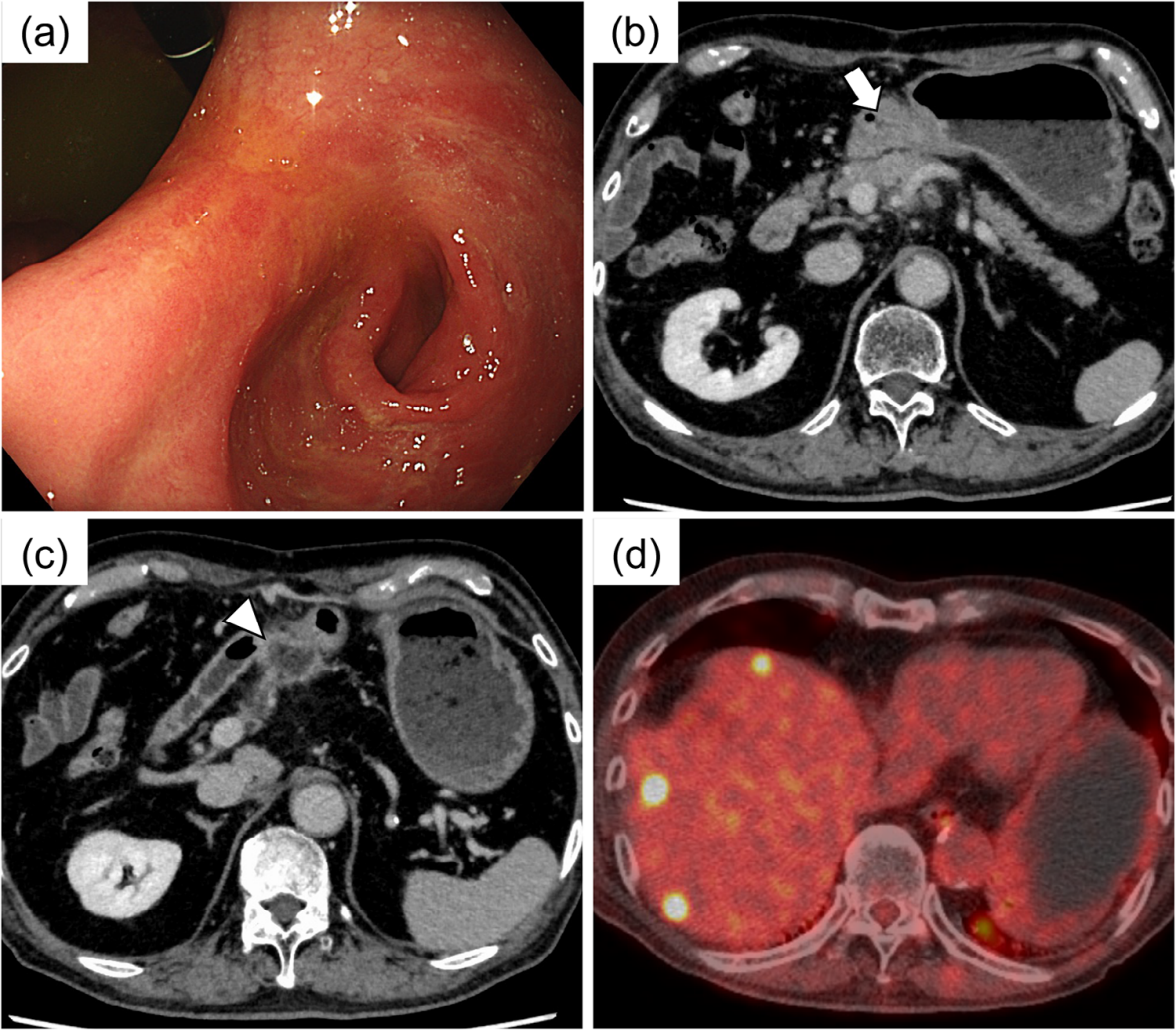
Endoscopic findings, computed tomography (CT), and 18F‐fluorodeoxyglucose positron emission tomography (FDG‐PET)/CT images. (a) Esophagogastroduodenoscopy (EGD) showing a singular ulcer scar. (b, c) CT revealing wall thickening of the gastric antrum (white arrow) and an irregular mass on its dorsal side (white arrowhead). (d) FDG‐PET/CT showing FDG uptake in the liver.
